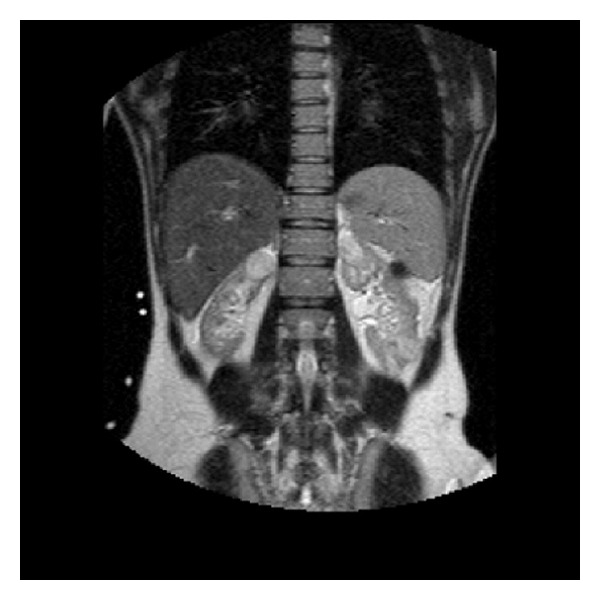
T2-W coronal MR image demonstrates a large right renal mass and numerous left renal masses, some of which were not evident on the US.
